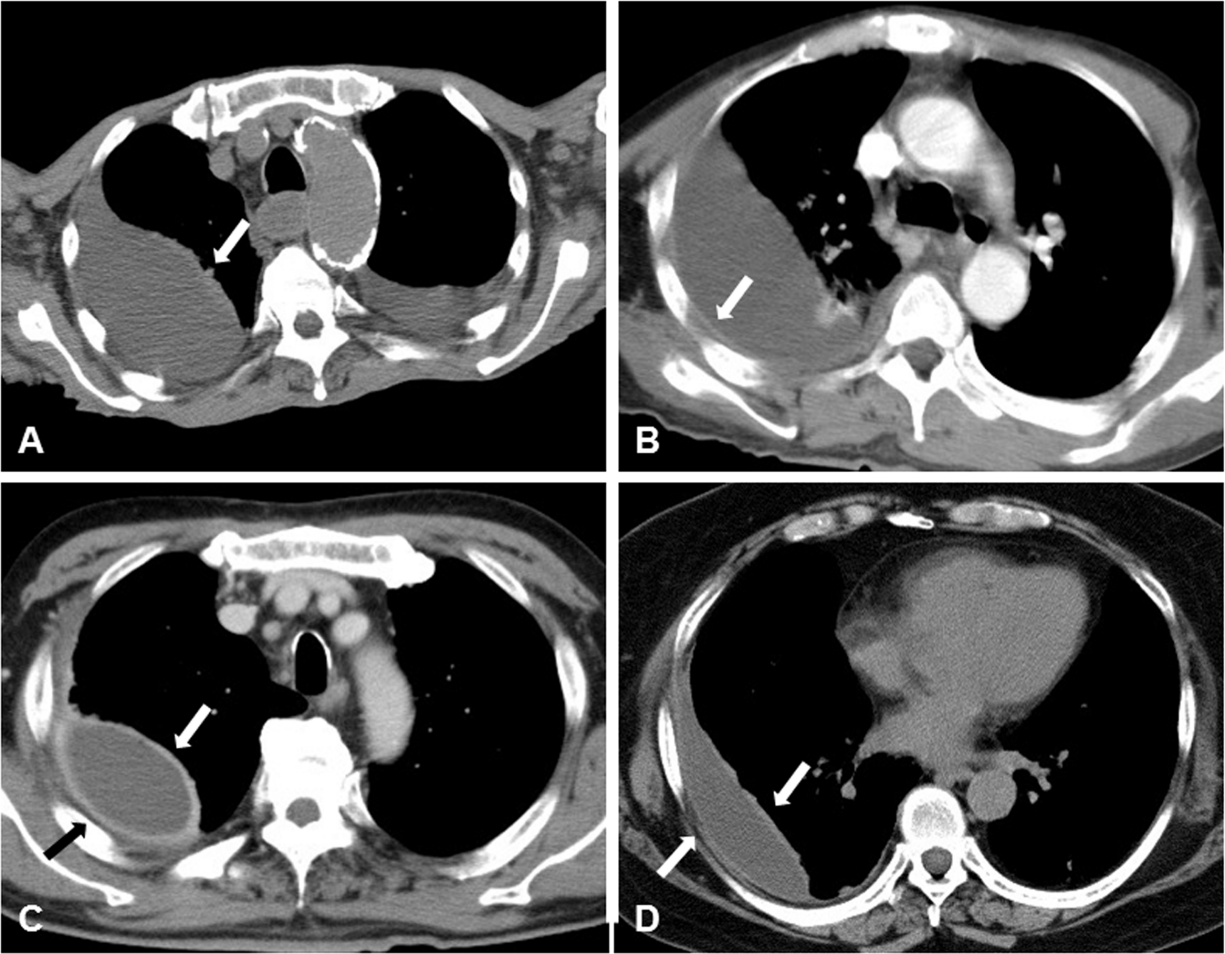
Representative figures for hemi-split pleura sign (A, B) and split pleura sign (C, D). Thickened visceral or parietal pleura on thoracic CT show the “hemi-split pleura sign” (A, B). When both pleura are thickened and separated by effusion, this is defined as the “split pleura sign” (C, D).
